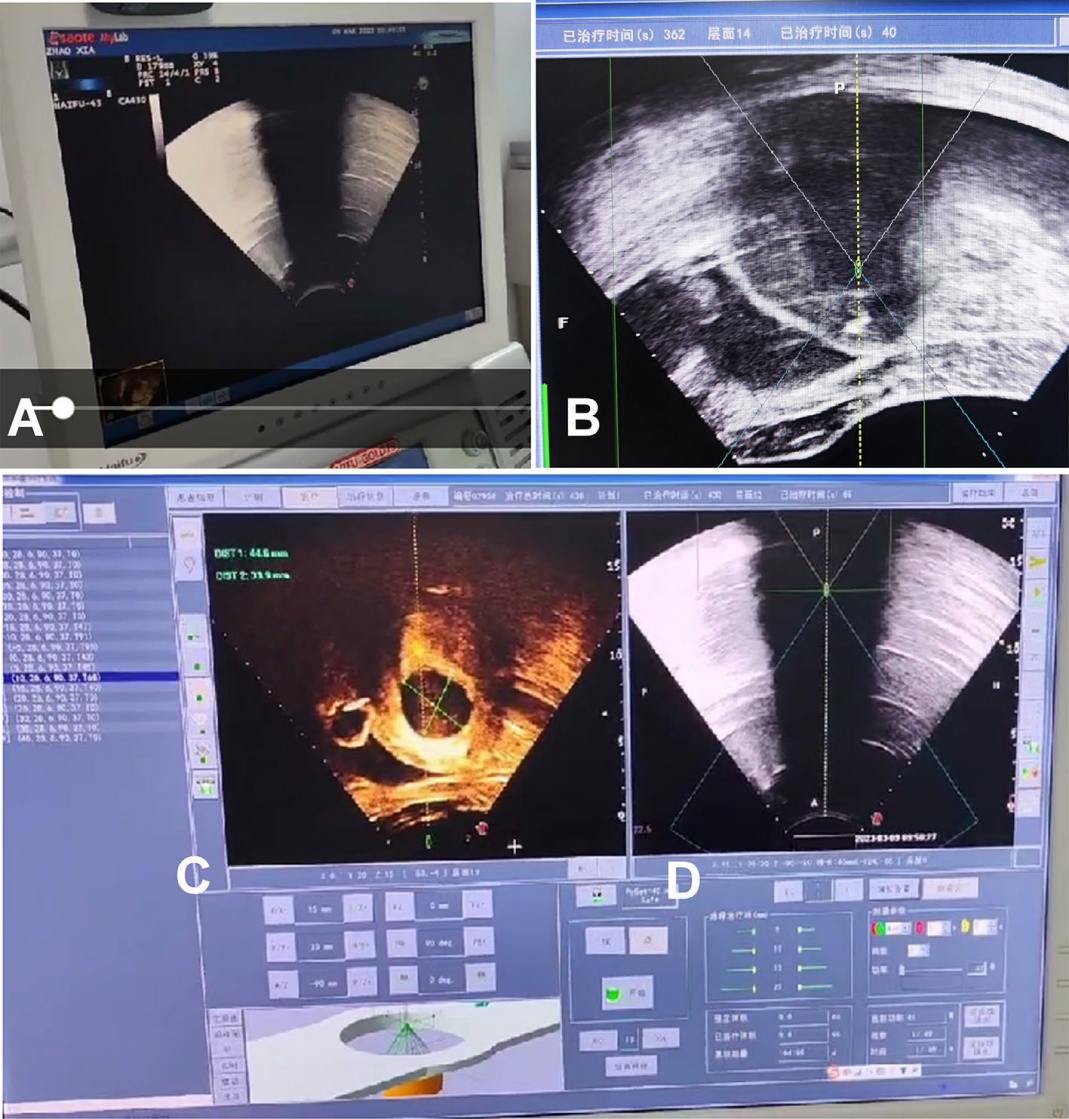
Real-time ultrasound monitoring of treatment of uterine ademomyosis with high-intensity focused ultrasound (HIFU). (A) Imaging of real-time ultrasound monitoring was displayed on the screen of one ultrasound scanner. (B) Real-time ultrasound monitoring showed that the total treatment time was 362 s, the treatment was on the 14th layer, and the treatment time on this layer was 40 s. (C) HIFU treatment imaging was displayed on the monitor screen of HIFU scanner. (D) The imaging of real-time ultrasound monitoring (A) was transferred to the HIFU screen (D) for real-time monitoring of the HIFU treatment effect, and if the treatment caused significant gray increase in the whole lesion area, the HIFU treatment would be stopped.
